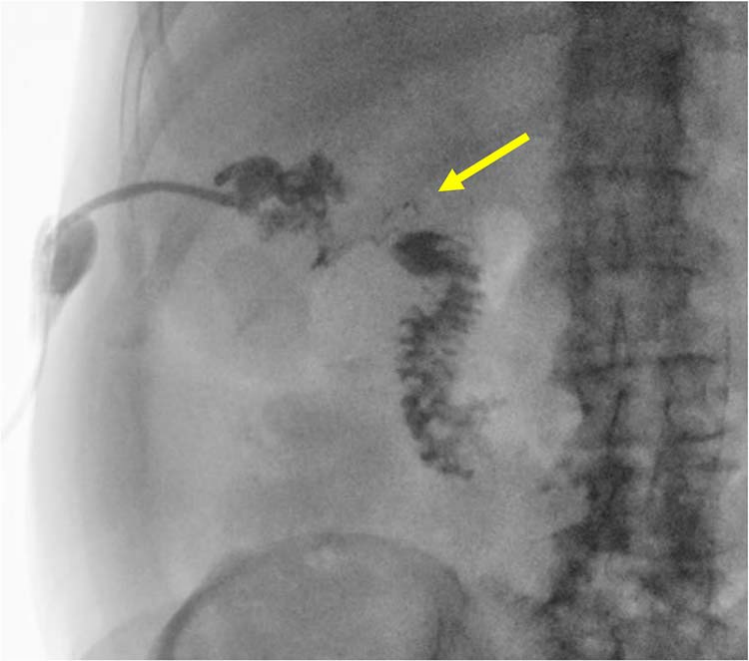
A radiographic fistulogram demonstrating the fistulous tract communicating with the second part of the duodenum.
